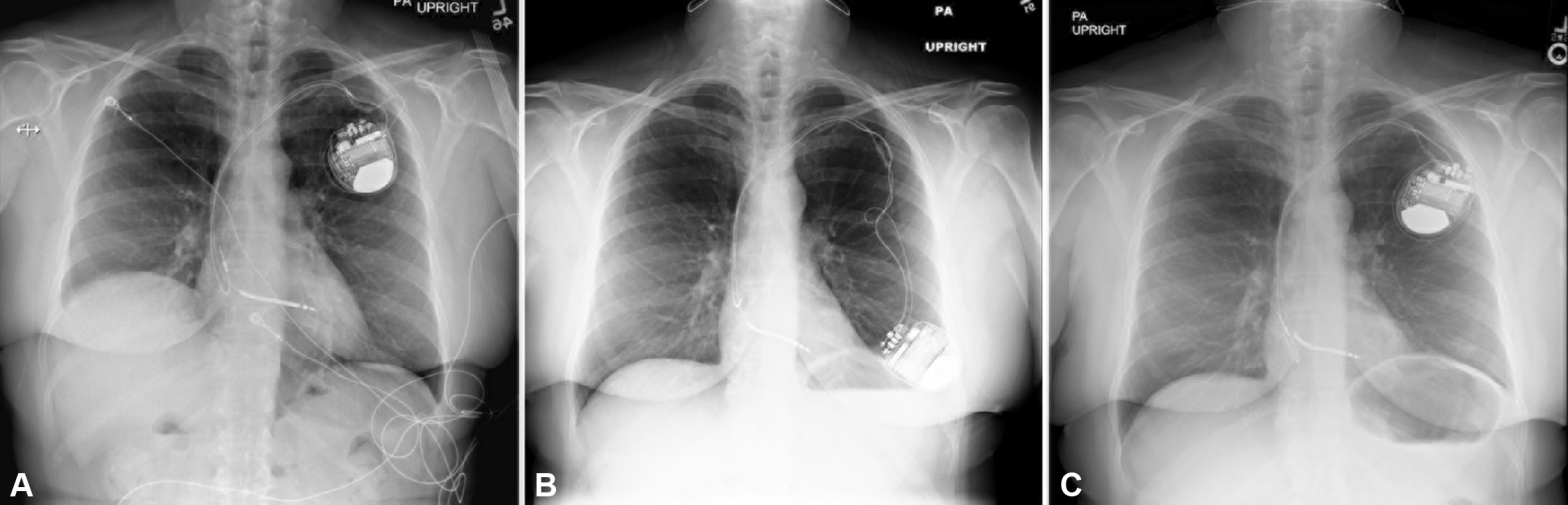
Poster-anterior (PA) X-ray images of the case patient. (
